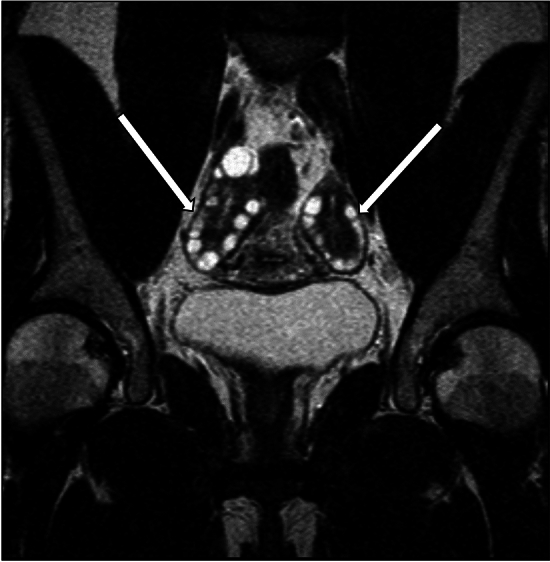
T2-weighted coronal MRI of the pelvis of a 29-year-old woman who presented with hirsutism and right ovarian cyst on ultrasound. Enlargement of both ovaries, which contain numerous peripheral small follicles in a “string of pearls” distribution, is seen (arrows). This appearance is compatible with PCOS
